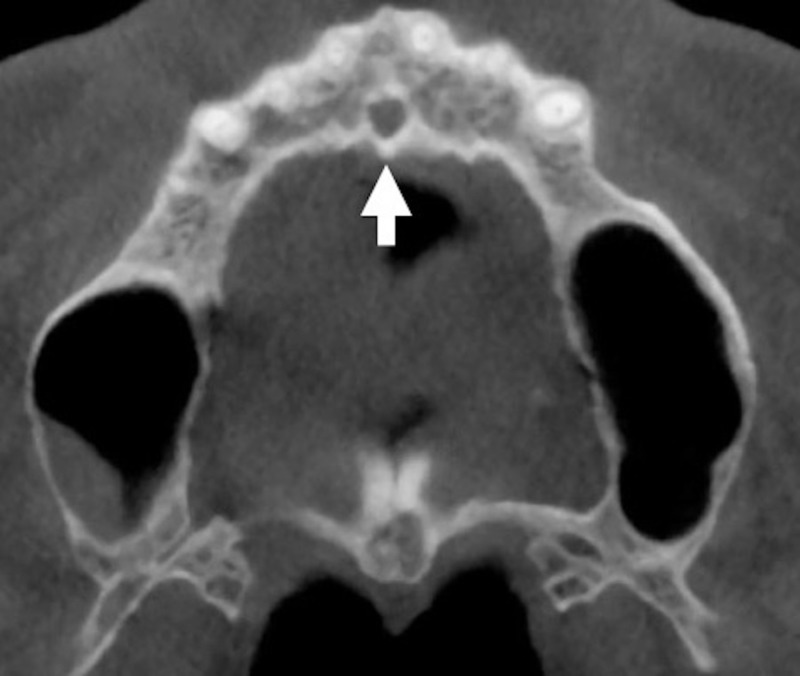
Axial plane of the incisive canal (arrow) on CBCT image. CBCT: cone-beam computed tomography
